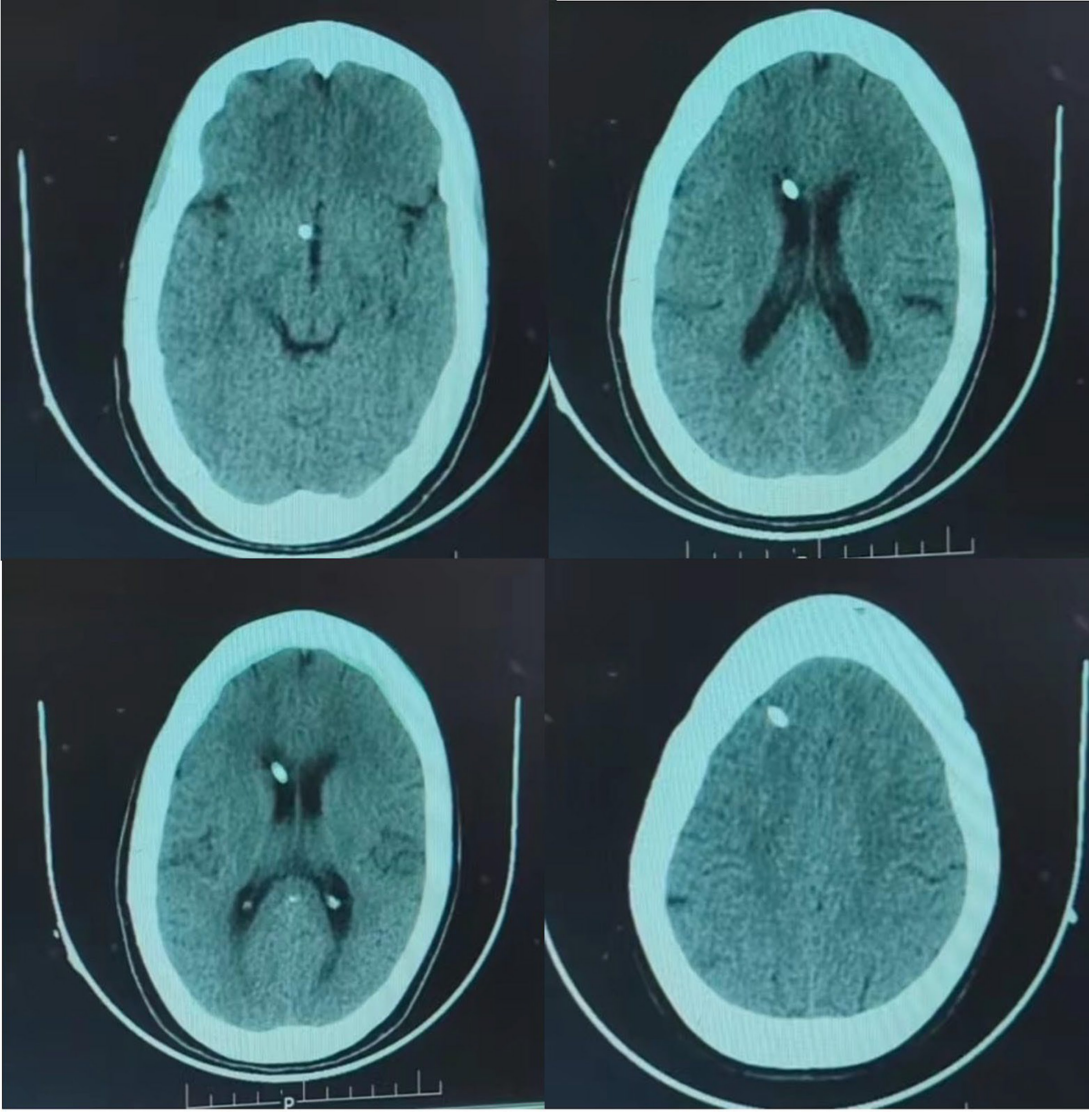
Axial sections of CT brain of our patient showing potency of VP shunt 9 months post surgery.
